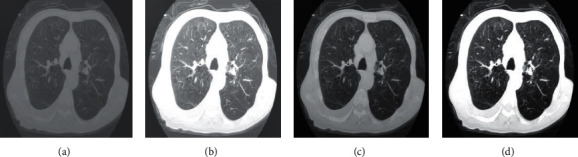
CT image processing effects of different algorithms. (a)–(d) the original image, CNN model processed image, ResNet model processed image, and DRCNN model processed image, respectively.
